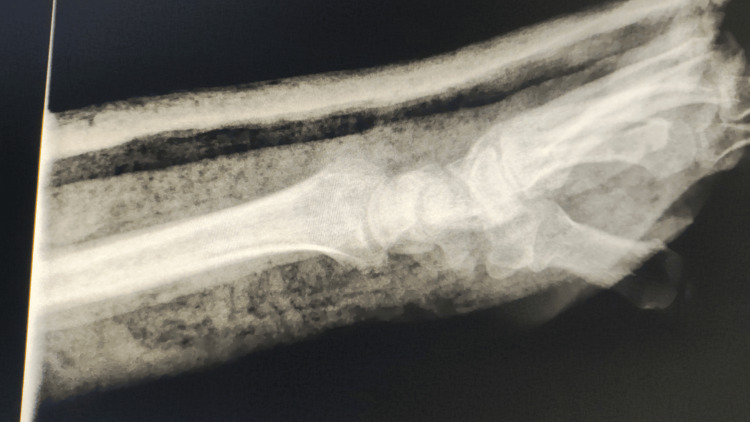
Post-reduction X-ray Post‑reduction lateral wrist radiograph confirming the anatomic reduction of the distal radioulnar joint.
